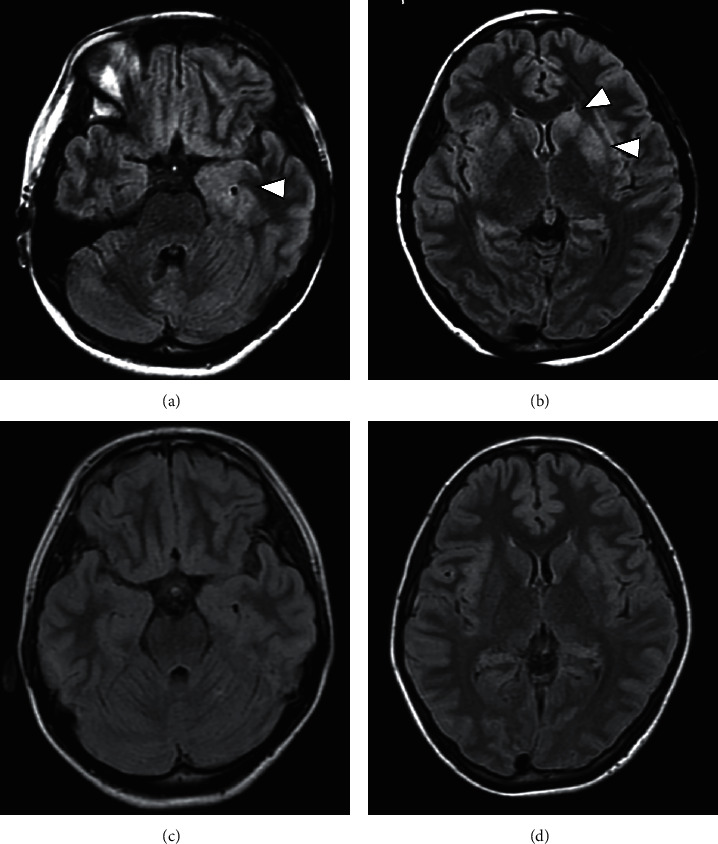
Brain MRI studies at the onset. Axial view of fluid-attenuated inversion recovery (FLAIR) images showing high-intensity lesions in the left medial temporal lobe (a), bilateral caudate nucleus, and left lentiform nucleus (b). (c), (d) MRI studies on the second day of follow-up. FLAIR images show a normal signal in the medial temporal lobe and basal ganglia and no abnormal lesion. Arrowheads indicate high-intensity lesions.
